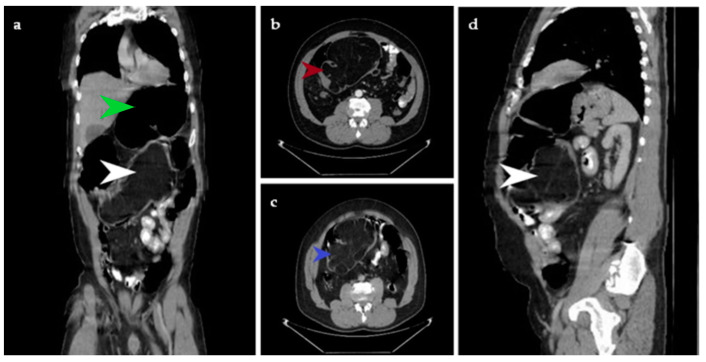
The CT examination with contrast substance reveals, in the coronal plane, voluminous lipoma with measurements of 16/11/12 cm projected in the lumen of the ascending and transverse colon without being able to establish the parietal affiliation. The tumor is indicated with a white arrow. The green arrow shows colonic dilation (a). A transverse section in arterial time reveals a voluminous lipoma, indicated with the red arrow (b). A cross section in venous time shows a lipomatous tumor, indicated with the blue arrow (c). In the sagittal plane, the same tumor is indicated with a white arrow (d). We present the case of a 46-year-old patient who arrives to the Brașov Emergency Department for rectal bleeding with fresh blood and blood clots which started about 12 h prior. The arterial blood pressure value was 100/50 mmHg, the heart rate was 100 bpm, and oxygen saturation (SpO_2_) = 96%. The patient had no medical family history. He was known to suffer from stage 2 hypertension, and he was a smoker and occasional alcohol user. All these symptoms appeared against a background of abdominal pain that debuted about 2 years ago, accompanied by intestinal transit disorders: constipation followed by diarrhea that relieves the pain. It was initially decided to hospitalize the patient at the Gastroenterology Clinic for investigations and specialized treatment. General clinical examination revealed pale and sweaty facies, and pale skin and mucous membranes. On examination, the abdomen was distended, mobile with respiratory movements, painful in the right iliac fossa, right flank and epigastrium and with no signs of peritoneal irritation. Rectal examination highlighted a normal-looking perianal region, normotonic anal sphincter, normal-sized prostate with preserved median groove and rectal ampulla that contained fresh blood and blood clots. The paraclinical examination detected Hemoglobin (Hb) 7.7 g/dL, Hematocrit (Ht) 21.6%, Serum iron test 34 ug/dL (normally 37–158 ug/dL). Colonoscopy was performed, and blood traces were detected in the colon and at 100 cm from the anal verge there was a large vegetative formation that occupied the entire lumen and could not be passed with the endoscope.
